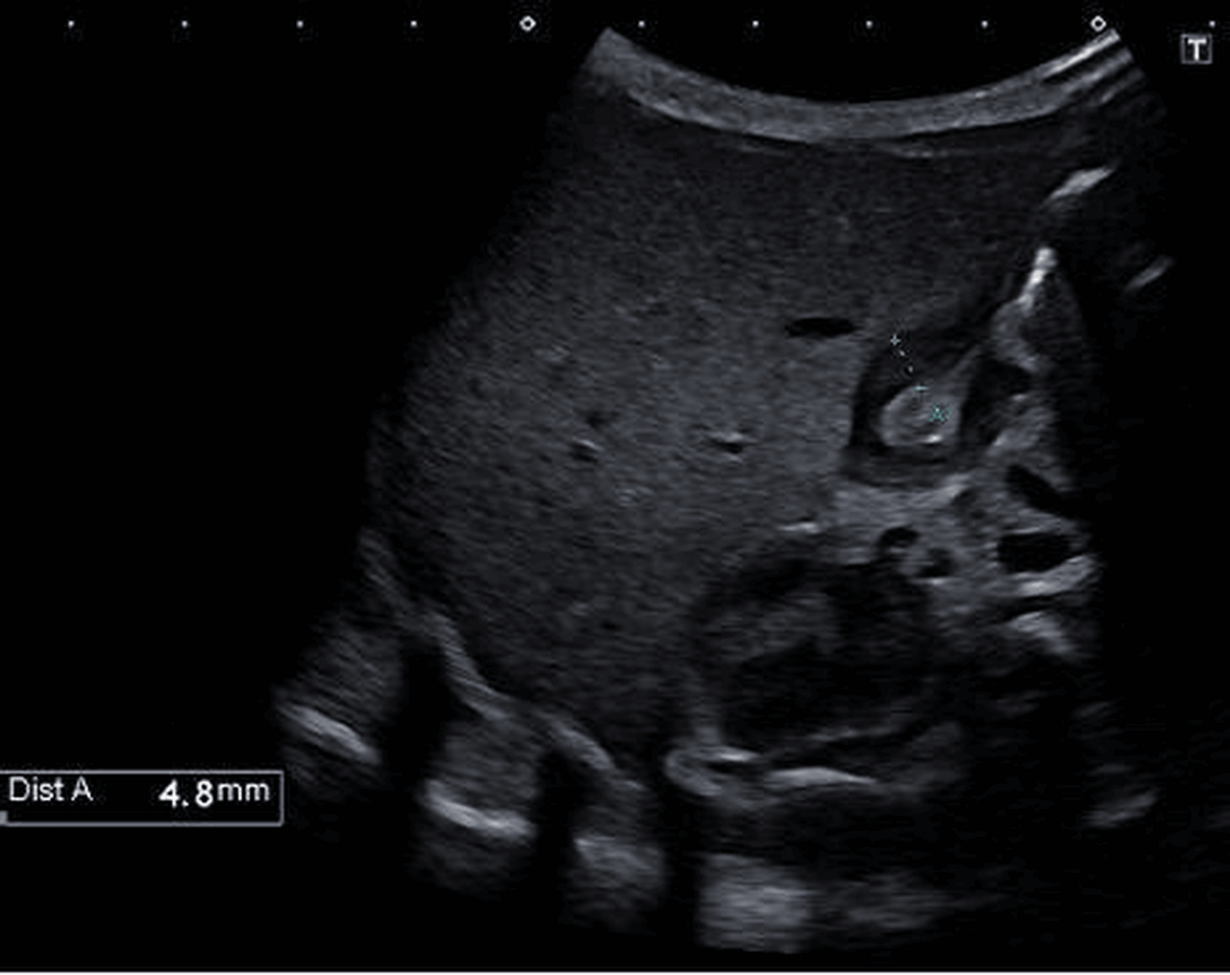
Ultrasonography showing a pyloric muscle thickness of 4.8 mm
